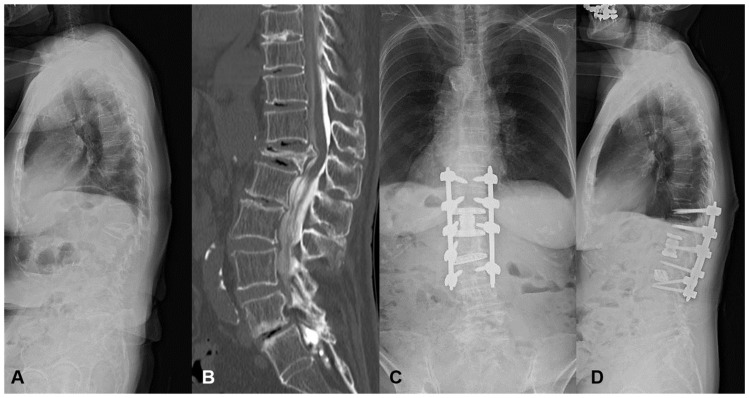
An 84-year-old female suffering from L1 vertebral collapse. The L1 MIS corpectomy, artificial body replacement, and L2/3 XLIF were performed followed by PPS fixation. The patient became ambulatory, and motor weakness recovered without mechanical complications. (A) Preoperative lateral X-ray, (B) Preoperative myelogram CT, (C) Standing AP X-ray at one year postoperatively, (D) Standing lateral X-ray at one year postoperatively.
